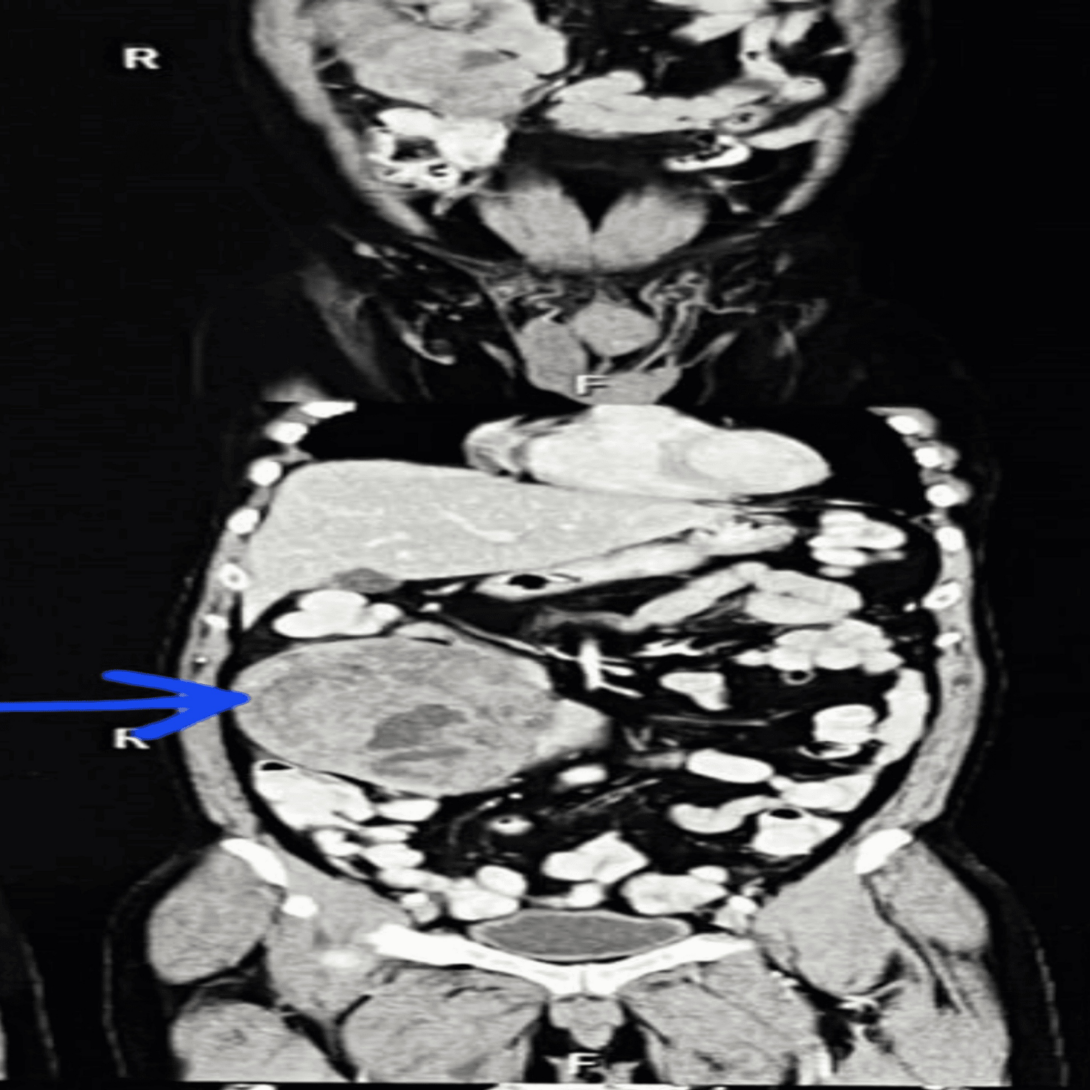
CECT of the abdomen A lobulated, heterogeneously enhancing soft tissue mass, most likely of small bowel origin (arrow). CECT: Contrast-enhanced computed tomography
